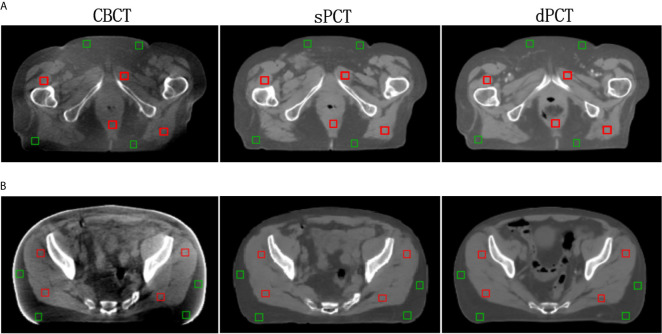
Distribution of the region of interests (ROIs). Two rows (A, B) represent the axial images of two patients respectively. The left, middle, and right images are CBCT, sPCT, and dPCT respectively. The green rectangle represents the fat ROIs, and the red rectangle represents the muscle ROIs. The corresponding ROI is placed in the same position on the CBCT, sPCT, and dPCT respectively. Each ROI contains 15 × 15 pixels. The display window is (700, 1300) HU.
